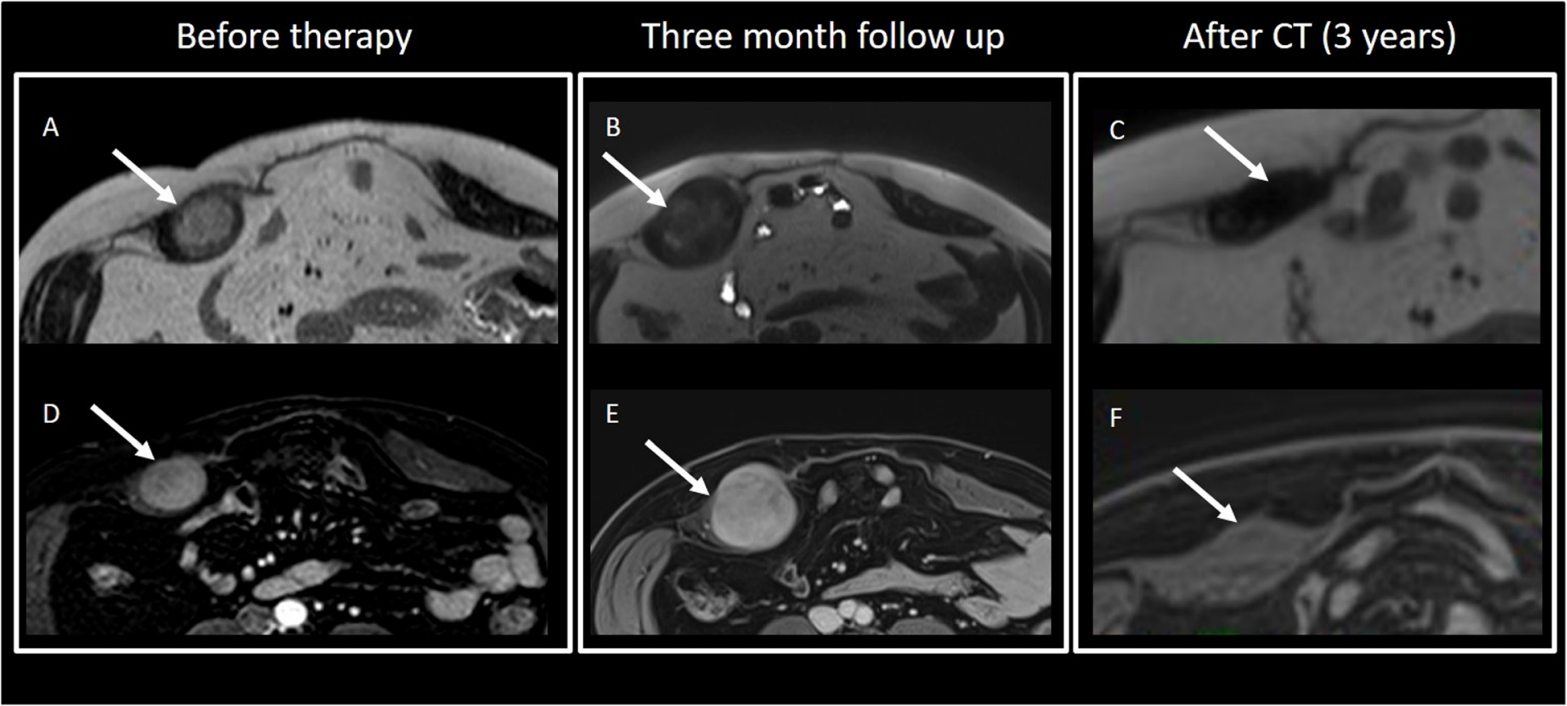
(Same patient of Fig. 16) Abdominal wall DT (T2wi in a and after contrast T1wi in b). Patient underwent to “wait and see approach” and then to CT therapy. MRI showed the variation of the signal on T2wi and of the contrast enhancement of the lesion (white arrow): after 3 months of follow-up, the lesion increased in dimension, maintained moderate contrast enhancement on T1wi (e), but became less hyperintense and more disomogeneus on T2wi. Then, the patient started a CT protocol with methotrexate and vinorelbine. After 3 years, the lesion significantly reduced its size, become strongly hypointense on T2wi (c), and did not show any significant contrast enhancement (f)
